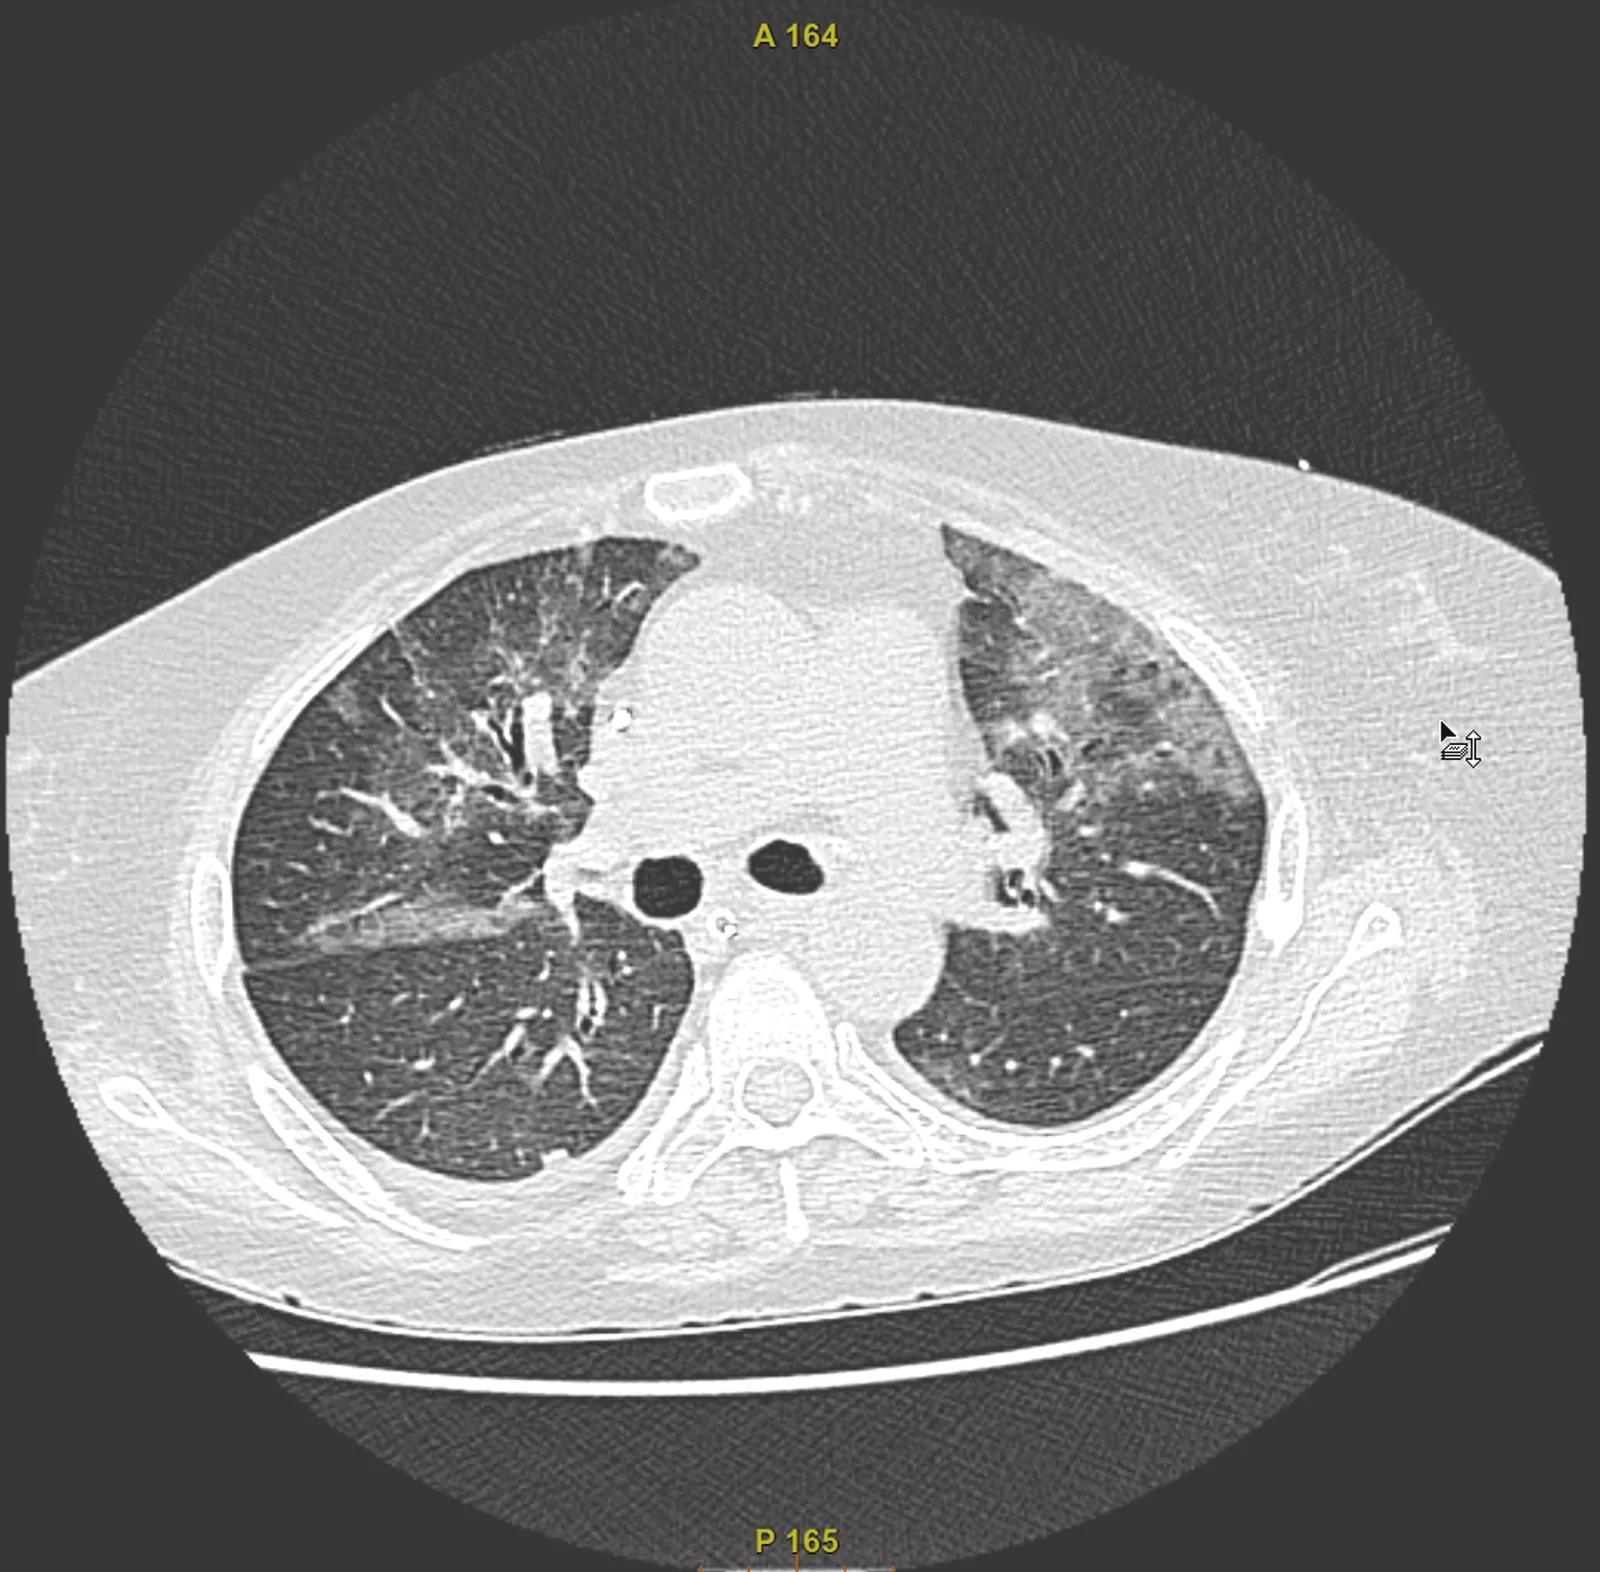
High-resolution computed tomography of the thorax showing bilateral ground-glass opacities
